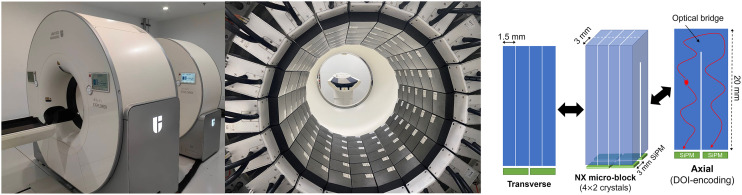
NX system. (Left) Overview of PET/CT with CT in front. (Center) NX PET detector ring (before removal of shoulder cutouts). (Right) U-shaped light-sharing detector array design providing DOI information.
